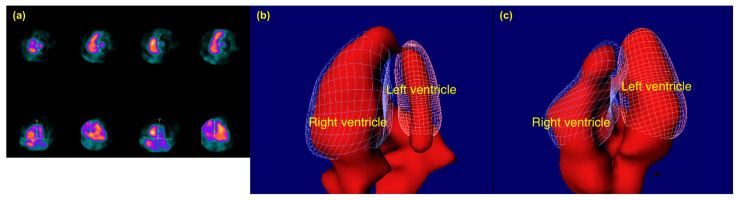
Representative end-diastolic CZT-SPECT-ERNA frames showing (a) two-dimensional adjustment mode, (b) dilated right ventricle, and (c) normal right ventricle as seen an apical four-chamber-like view. Images are representative of the assessment for RV dimensions in this study. In panel (a), colors are based on standardized uptake values confined by the color template (thermal), dotted lines indicate the region of interest, while solid lines with circles or squares denote anatomical landmarks such as the interventricular septum, tricuspid valve, and mitral valve.
